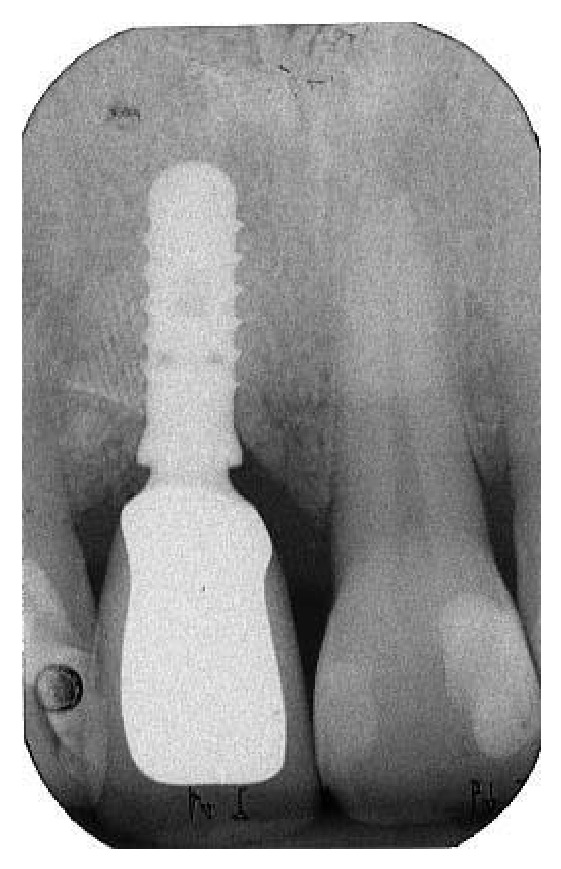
Periapical radiograph showing the definitive restoration seated in position.
